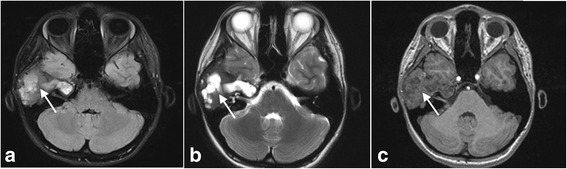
a Magnetic resonance imaging. T1-weighted image. b T2-weighted image. c Contrast-enhanced T1-weighted image shows a heterogeneous mass with contrast enhancement. The radiographic features of the lesion did not allow an experienced radiologist to establish the diagnosis
